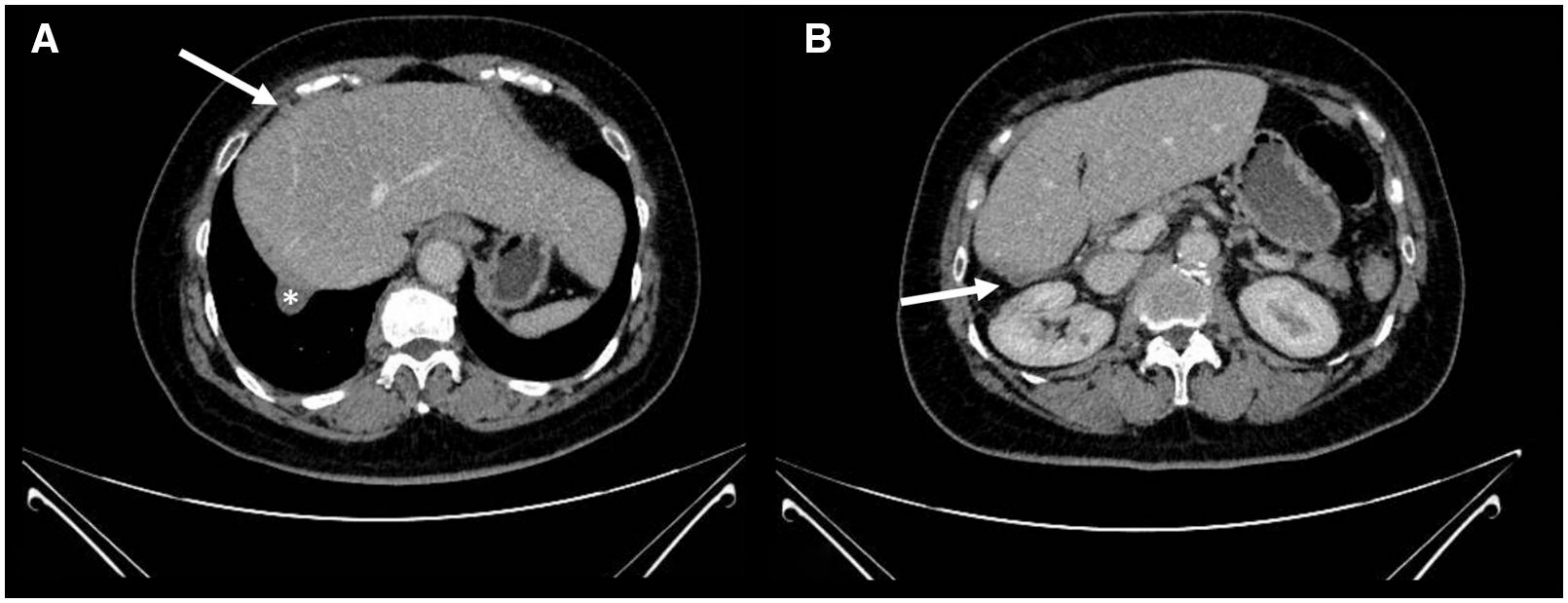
(A) Follow-up imaging (hepatic dome). CT-scan showing no recurrence in the treated area. The asterisk indicates the diaphragm. (B) Follow-up imaging (right Morrison pouch). CT-scan showing no recurrence in the treated area.
